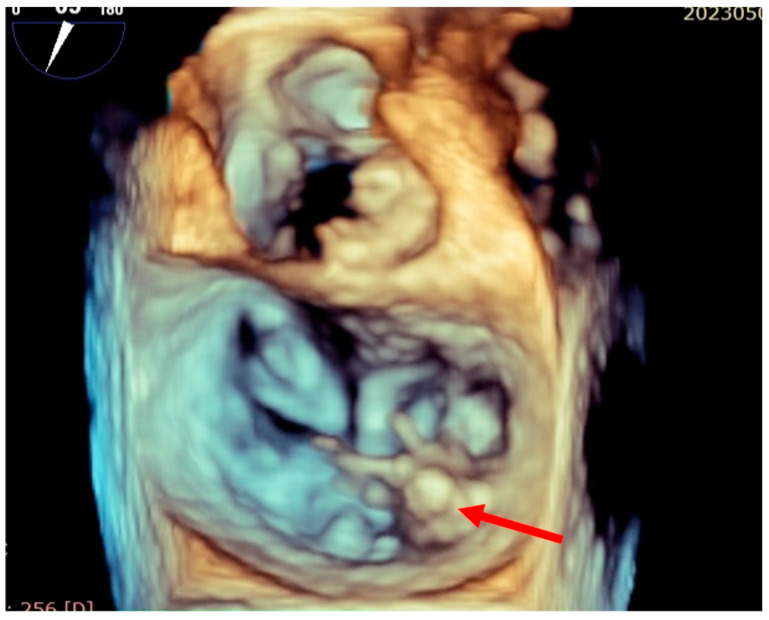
Intraoperative transesophageal echocardiography (3D reconstruction) revealing P3 flail due to chordae rupture (red arrow).
